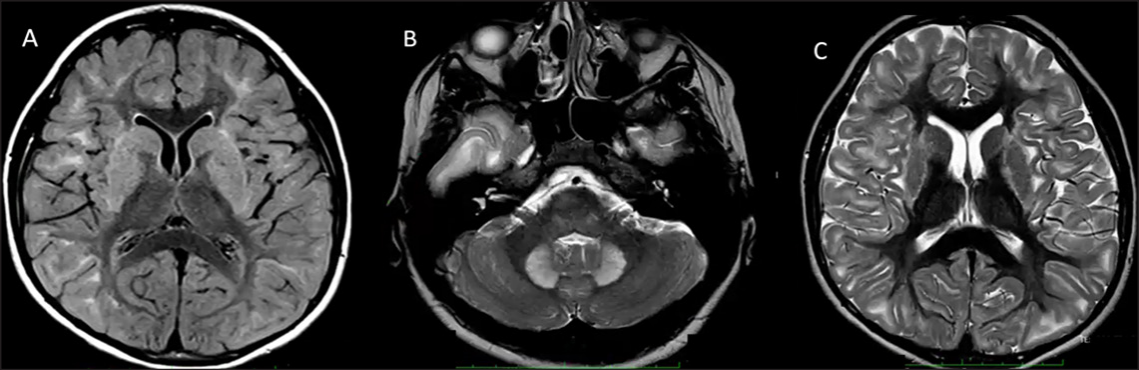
Axial brain MRI sequences. (A) FLAIR sequence shows bilateral subcortical white matter hyperintensities in frontal – parietal and temporal lobes. (B) T2WI with bilateral hyperintensities of dentate nuclei, and (C) T2WI showing bilateral hyperintensities in basal ganglia. Note in (A) and (C) the sparing of both thalami.
